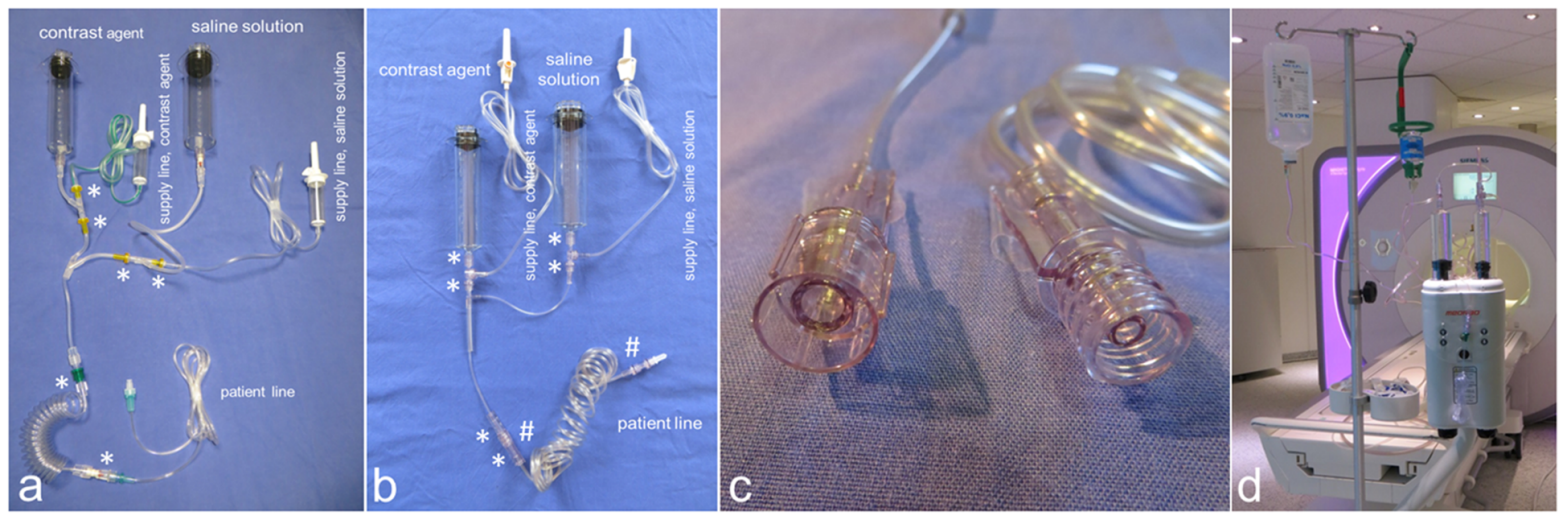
MRI tube systems Standard tube system (a) composed of a syringe for contrast media, a syringe for saline solution, a T-shaped connector tube with re-filling lines, a spiral infusion line, and the patient line. Novel tube system (b, d) composed of a syringe for contrast media, a syringe for saline solution (similar to a), a fluid transfer set for re-filling, a T-shaped connector tube, and a spiral patient line with shielded screw connectors (c; b #). Check walves within the tube system are marked by an asterisk (*).
